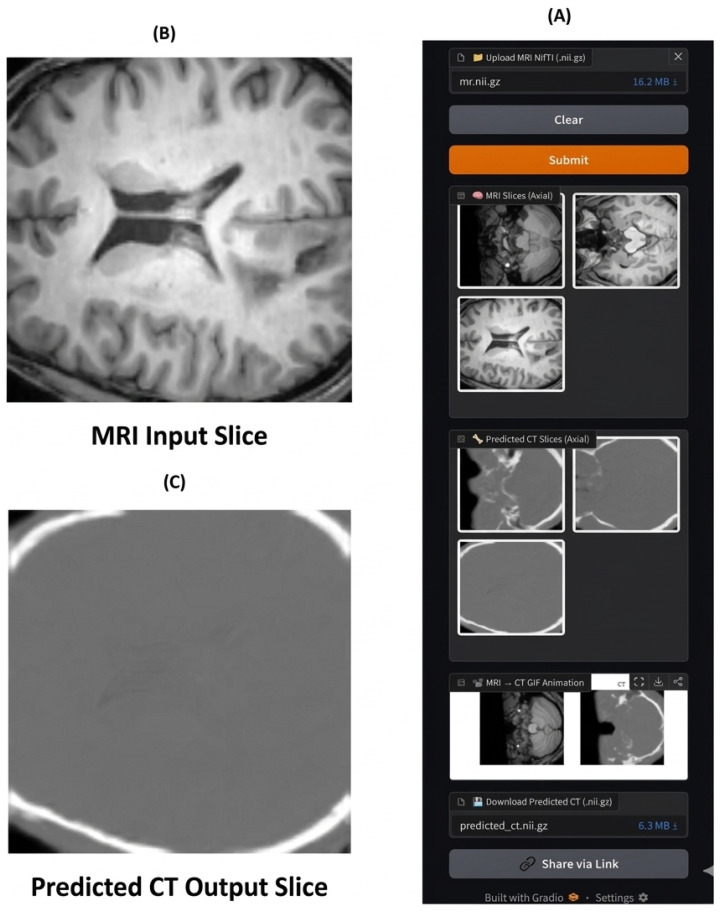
Overview of the Hugging Face deployment and inference demonstration of the improved 3D U-Net model for MRI-to-CT translation. (A) shows the interactive Hugging Face Space interface, including MRI upload, predicted CT visualization, and GIF animation features. (B) presents a sample slice of the MRI input used during inference. (C) shows the corresponding predicted CT slice, demonstrating the model’s ability to generate CT-like images from MRI data.
